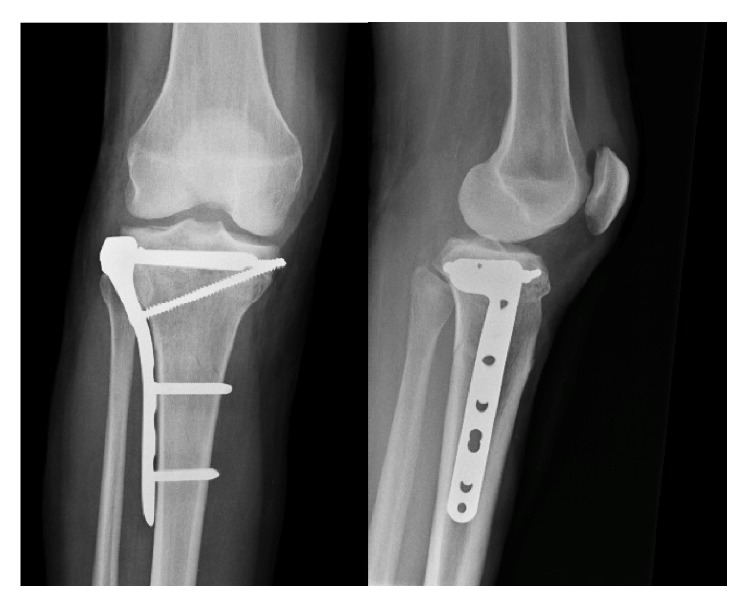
X-rays of the right knee after osteosynthesis.
